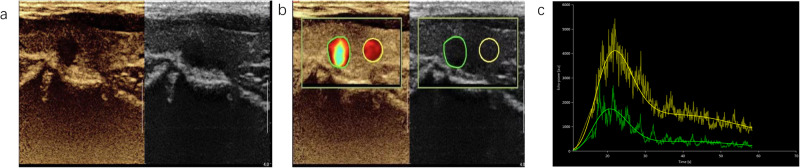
Contrast-enhanced ultrasound image of nodules (a). Quantitative analysis image of the left lobe nodule (b). TIC (yellow: normal thyroid tissues, green: nodules) (c)
